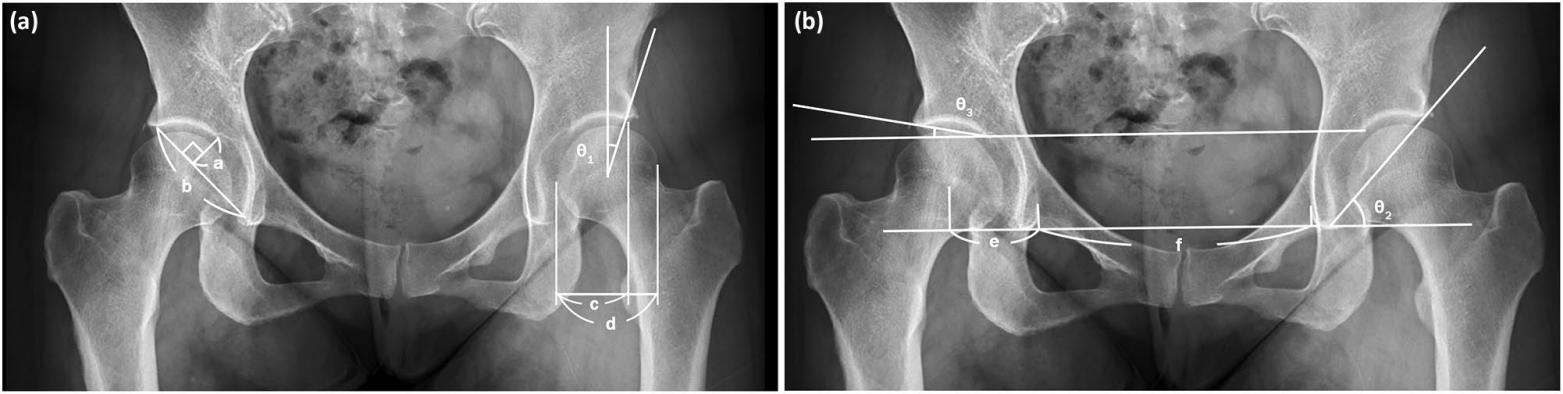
(A) Parameters used to measure acetabular dysplasia and lateral subluxation. θ1 is the lateral CE angle (LCEA). a/b is the acetabular depth ratio (ADR). c/d is the acetabulum head index (AHI). These parameters were measured on the AP radiograph. CE angle was measured as the angle between the line joining lateral aspect of the weight-bearing zone and the femoral head center and the line running vertically along the longitudinal axis of the pelvis. ADR was calculated by dividing the depth of the acetabulum by the length between the inferior teardrop point and the aspect of the weight-bearing zone, then multiplying by 100. AHI was calculated by dividing the length from the medial margin of the femoral head to the lateral rim of the acetabulum by the femoral head width, then multiplying by 100. (B) Parameters used to measure acetabular dysplasia and lateral subluxation. θ2 is the Sharp angle. θ3 is the acetabular roof obliquity angle of Tönnis (TA). e/(f*1/2) is the head lateralization index (HLI) described by Ninomiya S. These parameters were measured on the AP radiograph. Sharp angle was measured as the angle between the line joining the lateral aspect of the weight-bearing zone and the inferior point of teardrop and the line joining bilateral inferior points of teardrop. Acetabular roof obliquity angle of Tönnis was measured as the angle between the line joining the lateral aspect of the weight-bearing zone and the medial aspect of the weight-bearing zone and the line joining bilateral medial aspects of the weight bearing zone. HLI was calculated by dividing the length from the medial margin of the teardrop and the femoral head center by half of the length between bilateral medial margins of the teardrop
